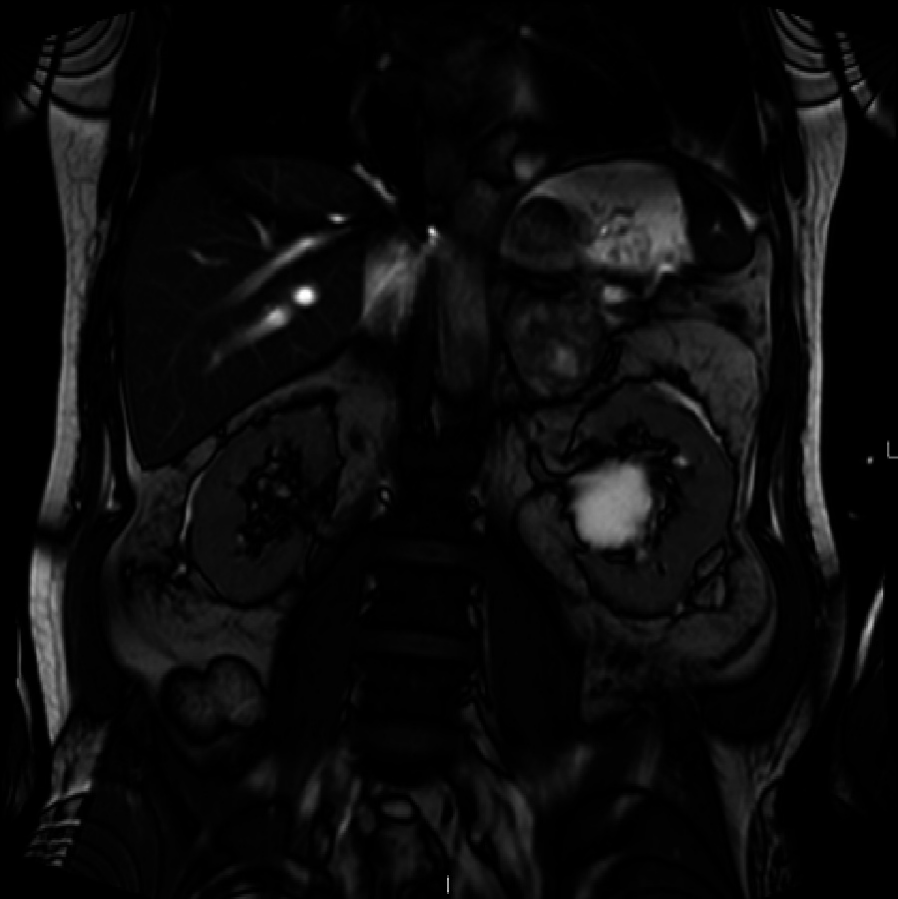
Computed tomography of the abdomen and pelvis Left heterogenous adrenal gland mass concerning for hemorrhage
